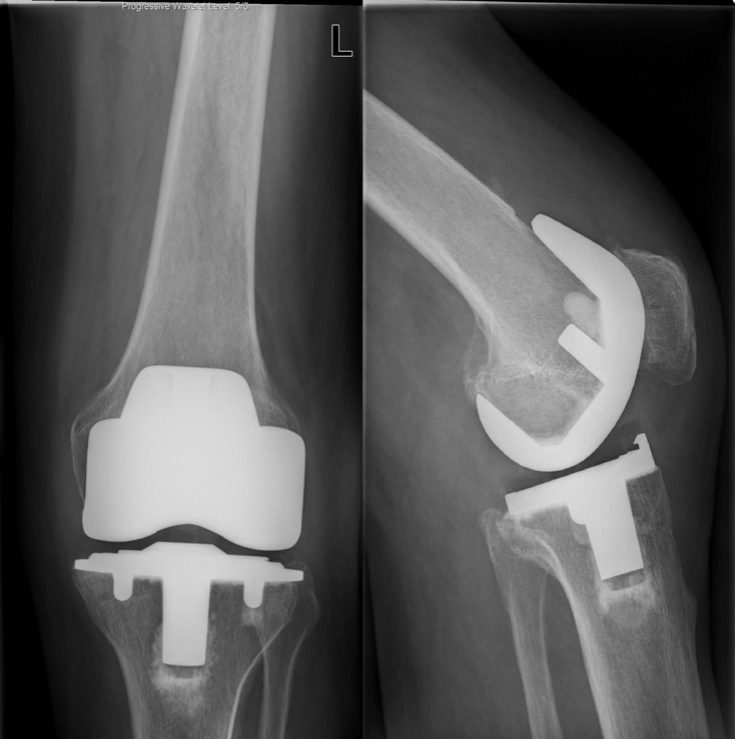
Radiographs of the prosthesis three years after total knee arthroplasty show normal alignment without evidence of loosening.
